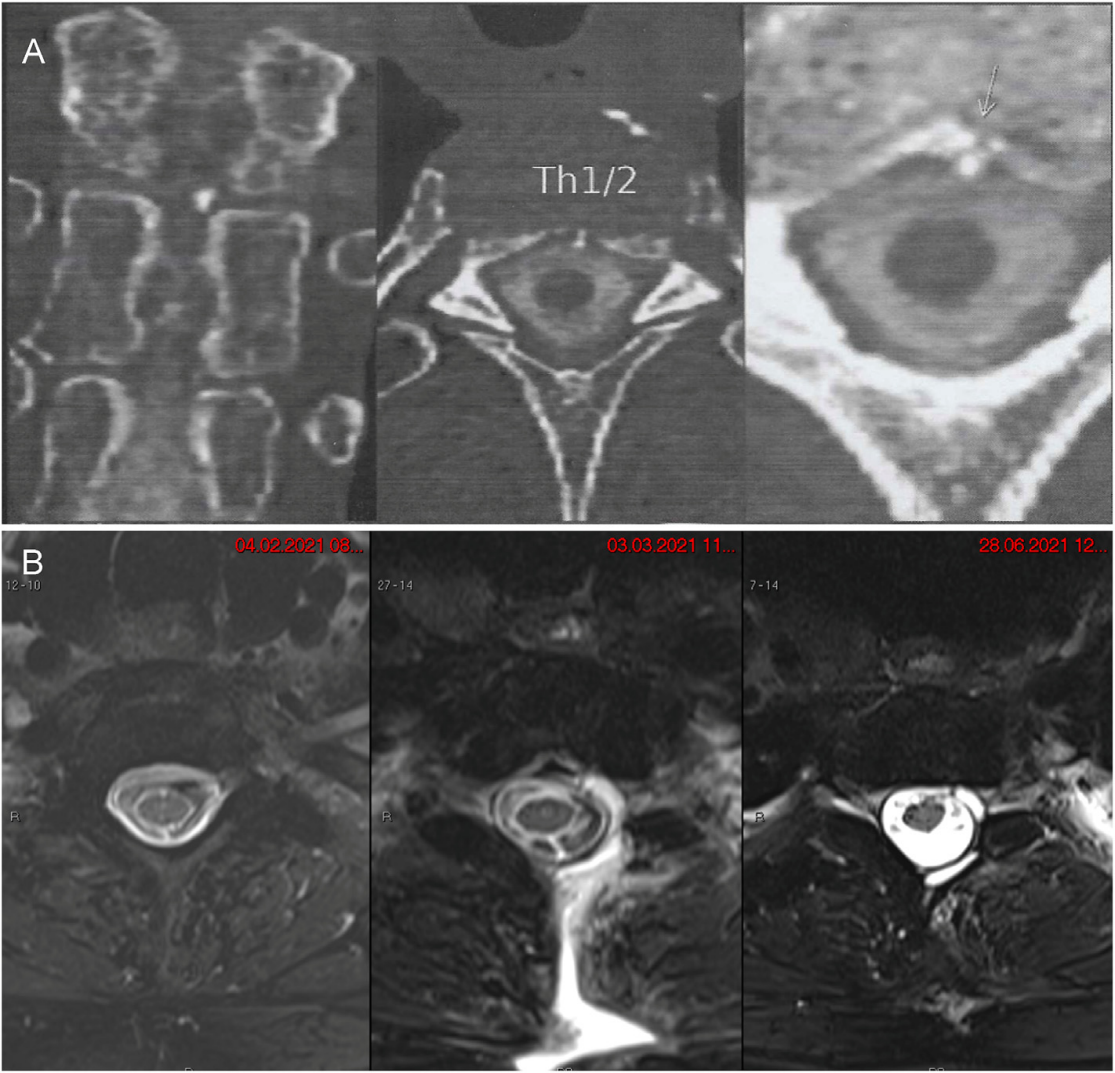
(A) CT myelography of the cervicothoracic spine four days preoperative, with a coronal and axial view of a DM out of the intervertebral disc at the T1-2 level, which was assumed to be responsible for the dural leak. (B) MRI of the cervicothoracic spine. (Left) Four days preoperative. (Middle) Three weeks postoperative with fluid extravasation at the T1-2 level. (Right) Five months postoperative with complete regression of the epidural leakage.
